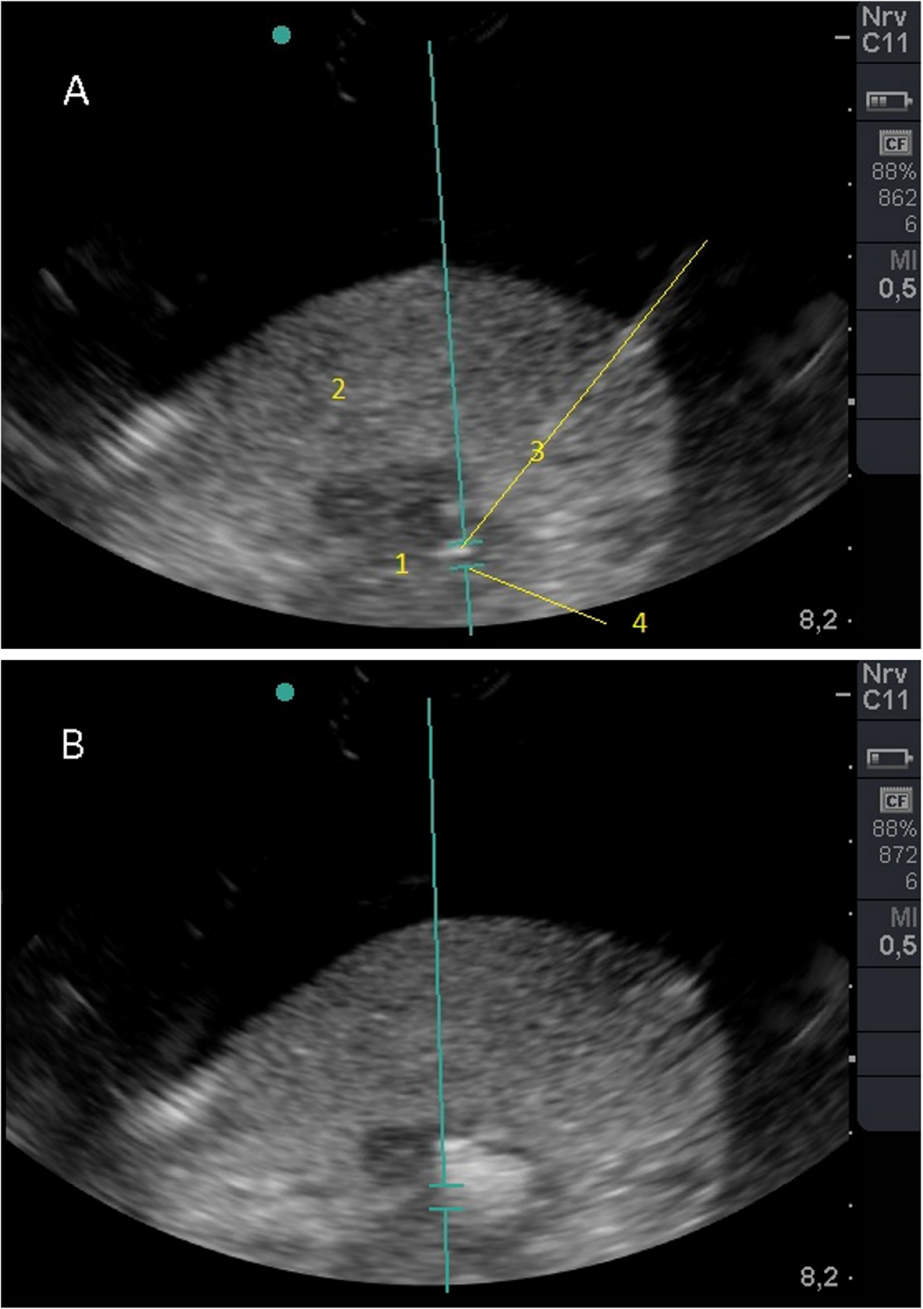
Sonomorphology of adenocarcinoma in *ex vivo *flooded human lung before and after high-intensity focused ultrasound. (A) B-mode ultrasound image of adenocarcinoma in flooded lung before *ex vivo* high-intensity focused ultrasound insonation. Adenocarcinoma (1), flooded lung (2), thermocouple (3), and focal zone of high-intensity focused ultrasound beam (4); (B) B-mode ultrasound image after single high-intensity focused ultrasound insonation demonstrates a sharply demarcated and strongly hyperechoic sonolesion within the tumour.
